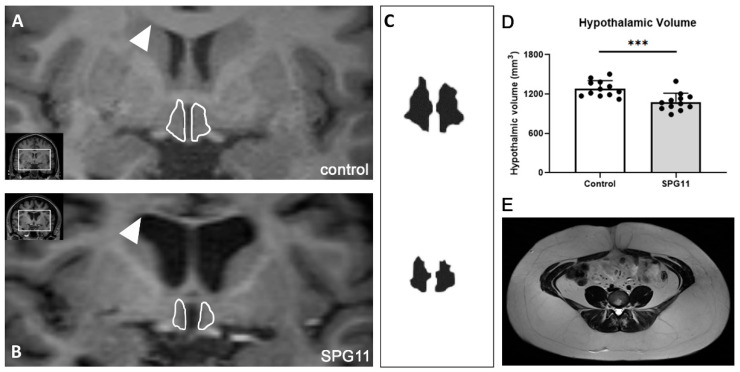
Reduced hypothalamic volume in SPG11. Representative images of hypothalamic volumetry with the MRIcloud software package in a control (A) and SPG11 patient (B). Arrowheads indicate the ventral border of the corpus callosum showing significant atrophy in SPG11. Outlines of the hypothalamus are in white lines, and 3D segmentation extracted from MRIcloud are shown in (C). (D) Quantification of hypothalamic volume in SPG11 and matched controls (n = 12). Bars indicate means ± SD. * *p* < 0.001. (E) T2-weighted axial image of abdominal adipose tissue obtained in a single SPG11 patient.
